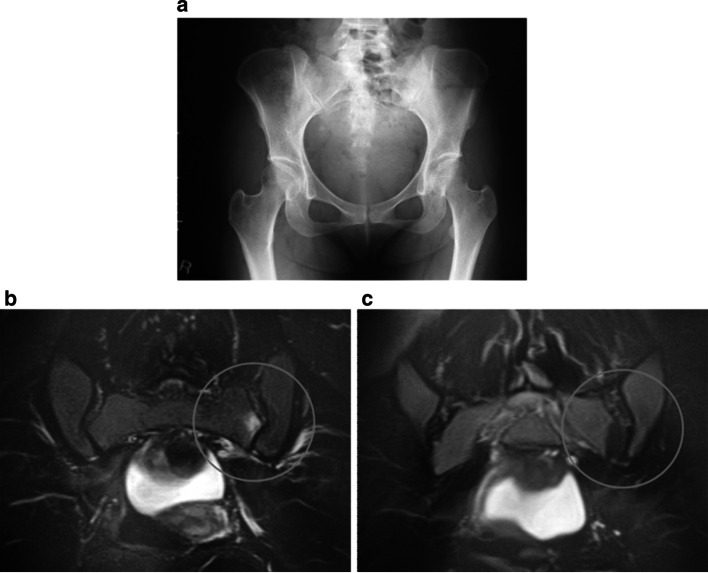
Radiography of the sacroiliac joints (a) and STIR sequence MRI for 1 patient with spondyloarthritis before (b) and after (c) treatment with adalimumab
